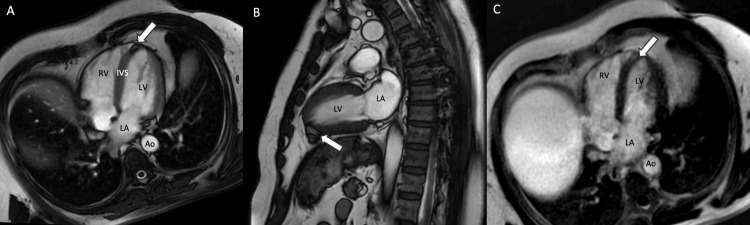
Four-chamber (A) and two-chamber (B) balanced steady-state free precession (b-SSFP) MRI images demonstrate a bright internal signal with a “black boundary” line at the interface of the lipoma within the myocardium. Four-chamber late gadolinium enhancement (LGE) image (C) shows a bright signal, which is nonspecific for infarct as fat (observe subcutaneous fat signal) or any other tissue with a short T1 time would also show a bright signal. Also, note the preservation of dark subendocardial signal adjacent to the fatty lesion. The constellation of findings from the b-SSFP images along with the CT confirms the fatty nature of the lesion rather than infarct-related LGE. RV: right ventricle; LA: left atrium; LV: left ventricle; Ao: aorta; IVS: interventricular septum.
